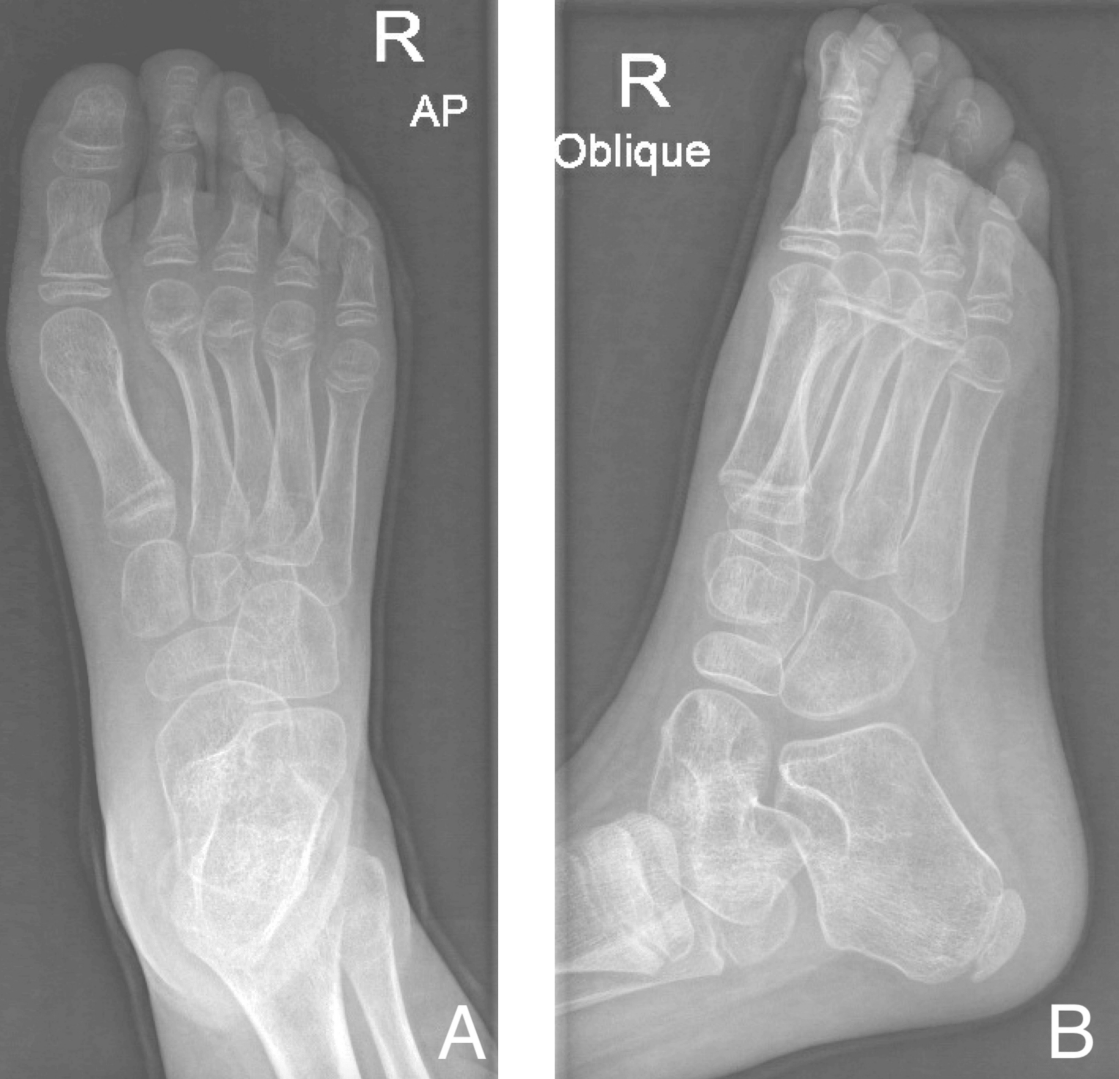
Postoperative X-ray of the right ankle Postoperative anterior-posterior (A) and oblique (B) X-ray of right ankle showing normal ankle alignment.
